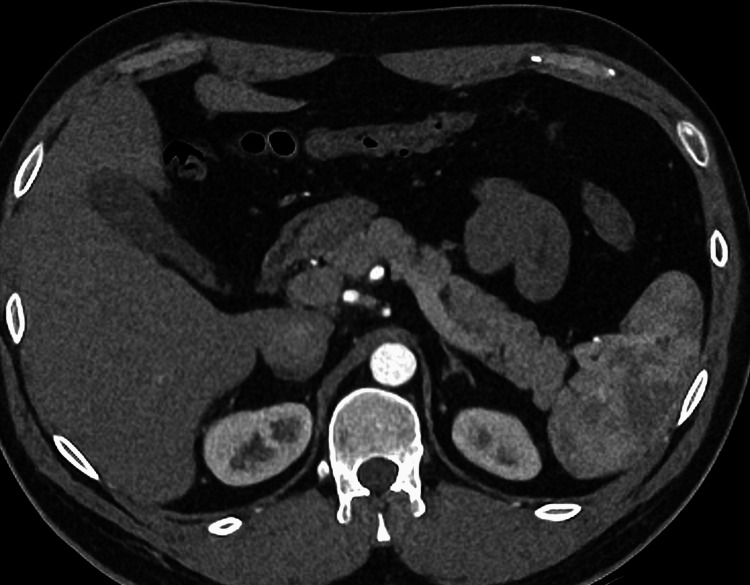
CT aortogram showing normal pancreas and gallbladder
